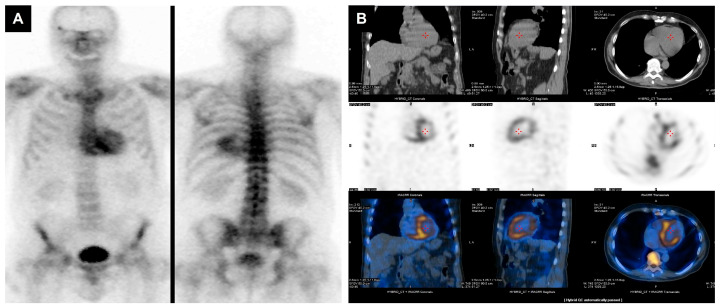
Planar and hybrid SPECT/CT bone scintigraphy in transthyretin cardiac amyloidosis. Anterior and posterior whole-body planar images (A) show intense myocardial tracer uptake exceeding rib activity, corresponding to Perugini grade 3 myocardial uptake, highly suggestive of transthyretin cardiac amyloidosis. Hybrid SPECT/CT images (B) in coronal, sagittal, and axial views confirm myocardial localisation of radiotracer signal, clearly distinct from blood pool or skeletal activity. The fused images (bottom row) demonstrate homogeneous radiotracer accumulation throughout the left ventricular myocardium, consistent with diffuse amyloid infiltration.
